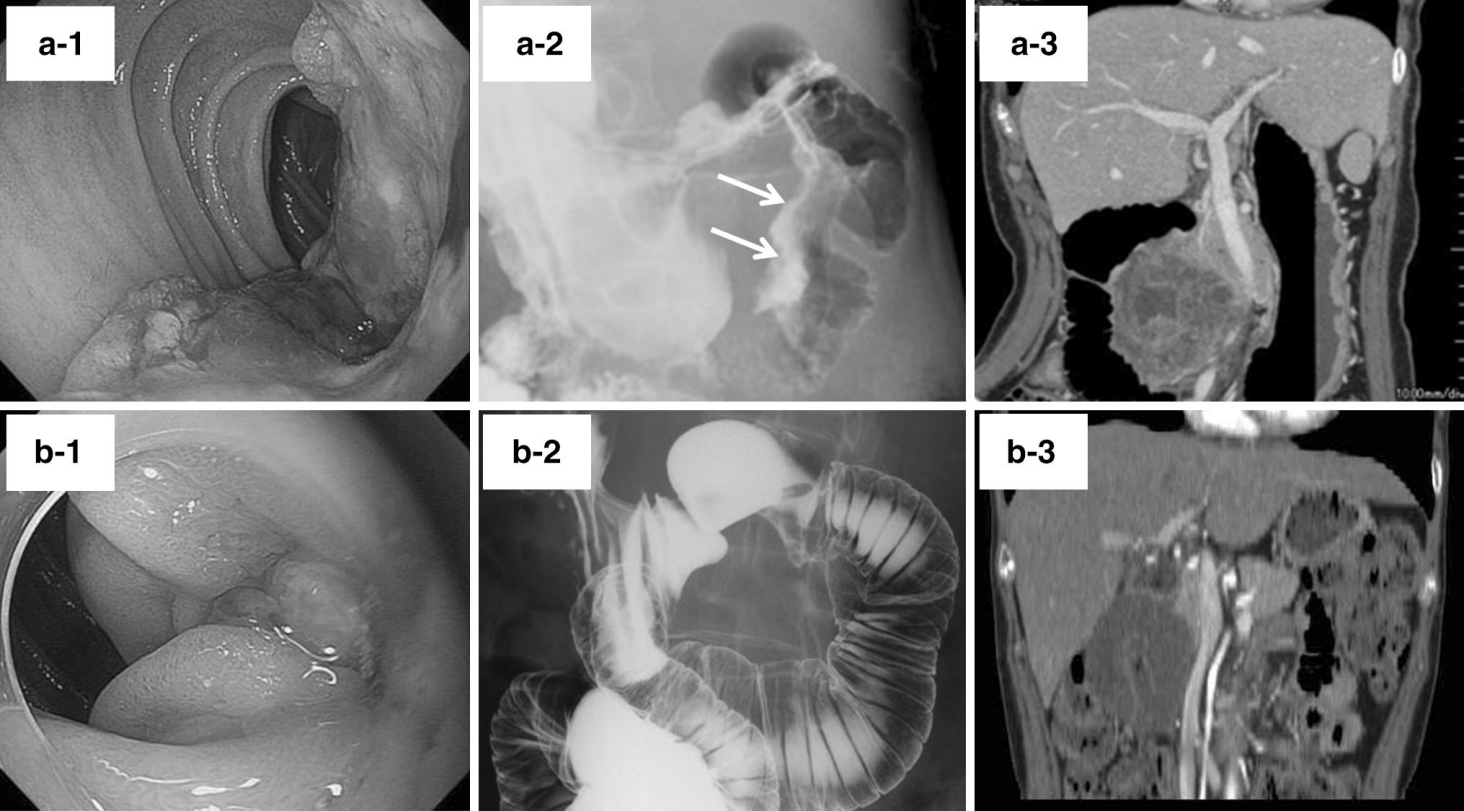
Imaging examinations. a Before chemotherapy. b After chemotherapy. a-1 Upper gastrointestinal endoscopy showing Borrmann’s classification type 2 cancer in the second part of the duodenum. a-2 Hypotonic duodenography showing the tumor (*arrow*). a-3 Abdominal contrast-enhanced computed tomography, showing a tumor 9 cm in diameter with invasion of the pancreatic head and superior mesenteric vein (SMV). b1-3 After chemotherapy, the tumor had decreased in size and invasion of the SMV had regressed
